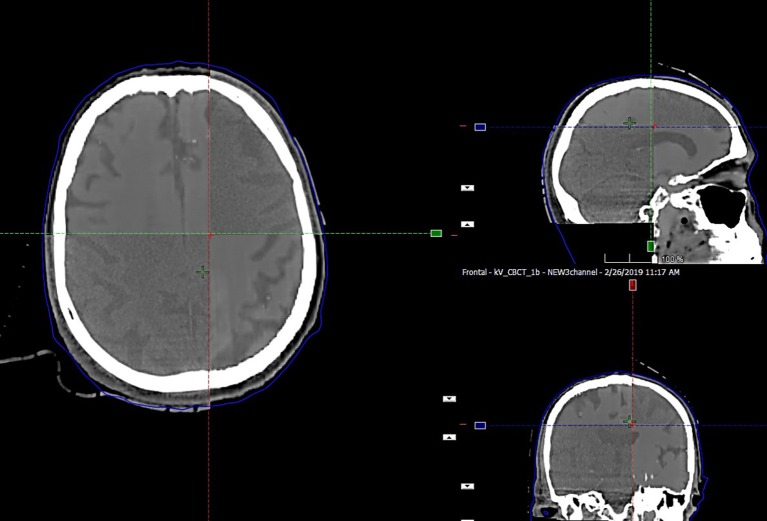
Split window display of MRCT aligned with CBCT for an example patient, demonstrating the preservation of dominant soft tissue interfaces such as major sulci and ventricles as seen in axial, coronal, and sagittal cross sections through the image volumes.
